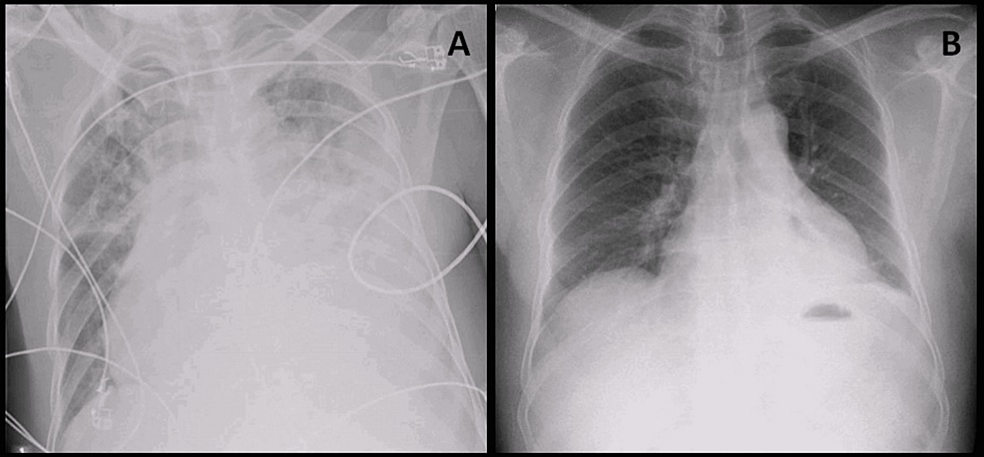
Imagiological evolution A: Admission chest X-ray; B: chest X-ray 2 months after finishing treatment.
